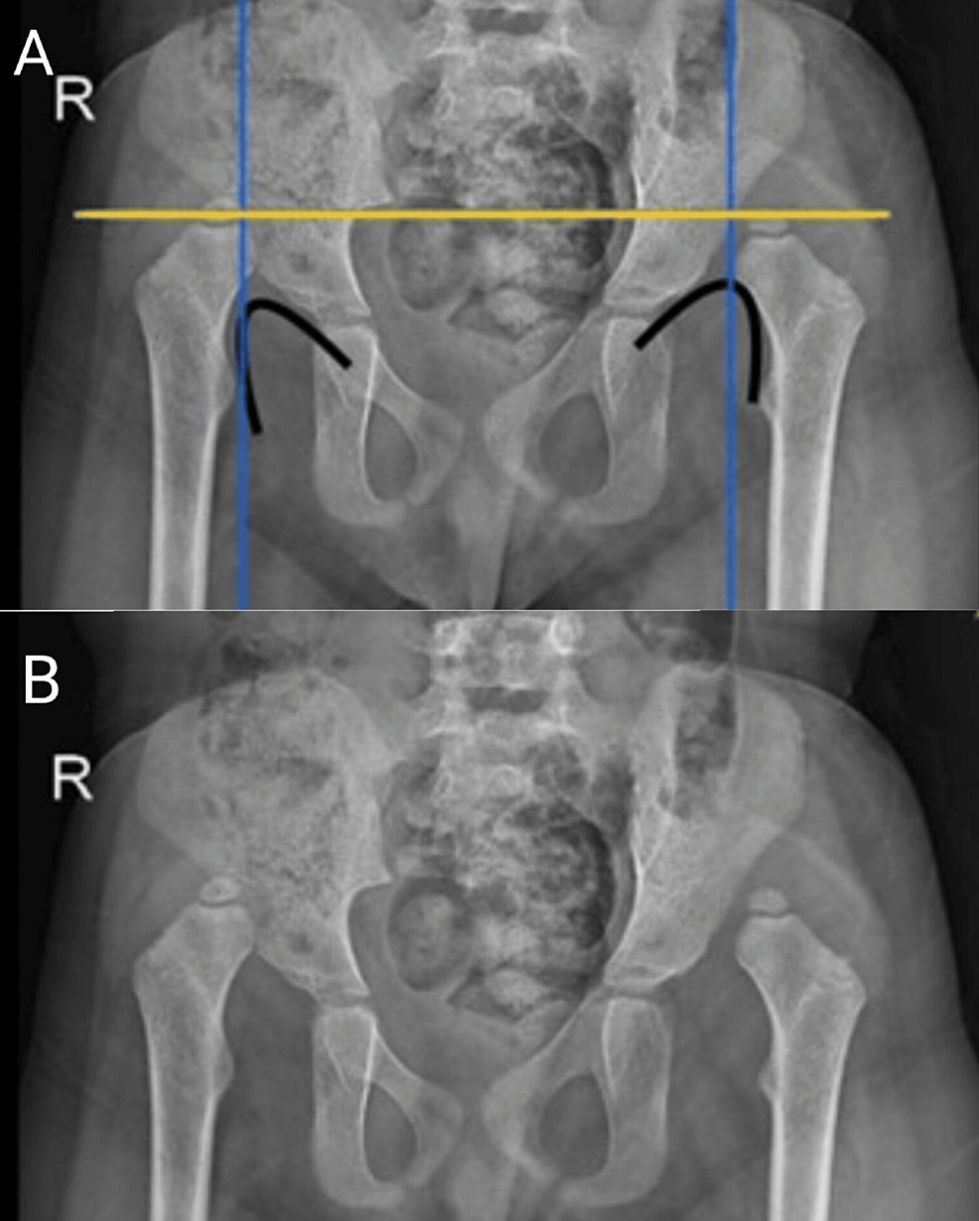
X-ray findings (A) shows a positive Shenton line in black, Perkins line in blue, and Hilgenreiner line in yellow; (B) shows developmental dysplasia of the hip
